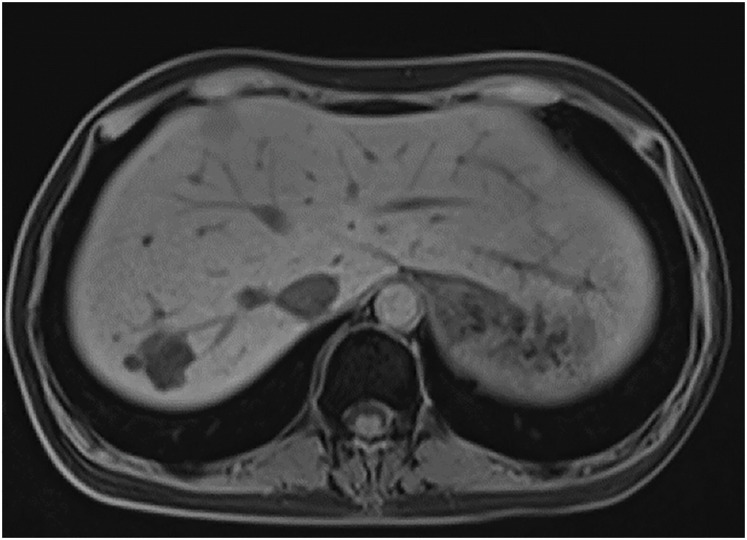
Late-phase Gd-EOB-DTPA-enhanced MRI of the S4 metastatic liver tumor after 10 courses of modified FOLFIRINOX chemotherapy, indicating a favorable therapeutic response. Gd-EOB-DTPA, gadolinium ethoxybenzyl diethylenetriamine pentaacetic acid
